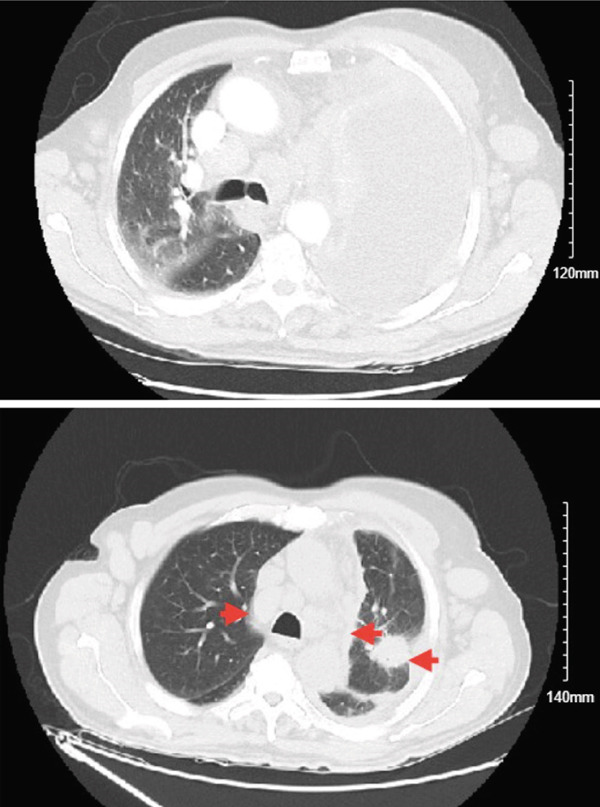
Chest CT showed a large left pleural effusion and revealed bulky bilateral axillary, right paratracheal, subcarinal, and right hilar lymphadenopathy. A left upper lobe mass of 3.3 × 4.3 cm was revealed after drainage of pleural effusion. Red arrows on the right are pointing to the borders of the left upper lobe mass, red arrow on the left is pointing to the bulky right mediastinal adenopathy.
